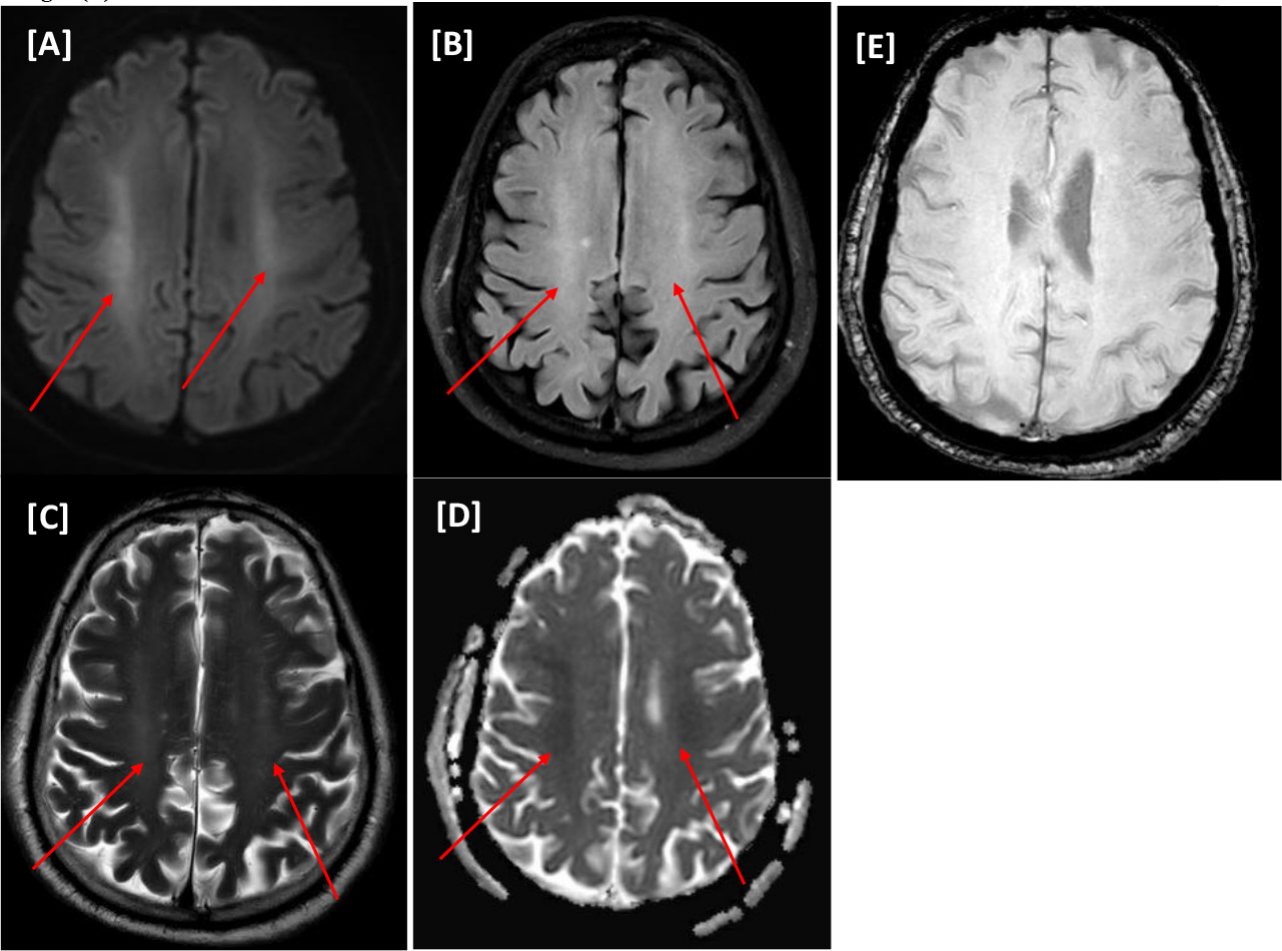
(Case VI) [A–E images] Magnetic resonance imaging of the head. Patchy rather symmetrical areas of bright signal intensities on T2WI/FLAIR involving the white matter of bilateral centra semiovale (A and B) with corresponding mild restriction patterns on diffusion-weighted imaging series of faint bright signals on b1000 and low value on apparent diffusion coefficient map (C and D). No microhemorrhage on susceptibility-weighted images (E).
